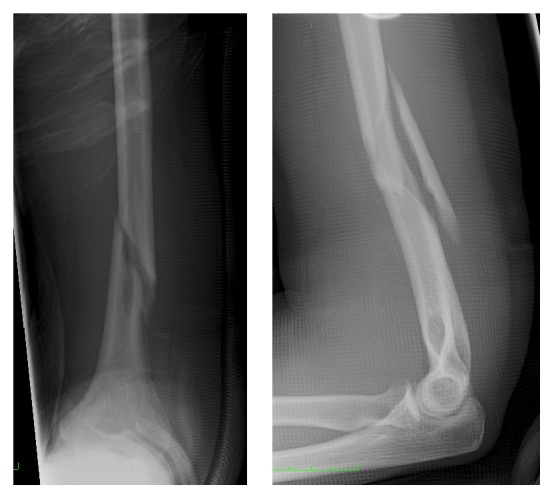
Preoperative anteroposterior and lateral radiographs of the slightly displaced distal humeral shaft fracture with a long wedge fragment.
